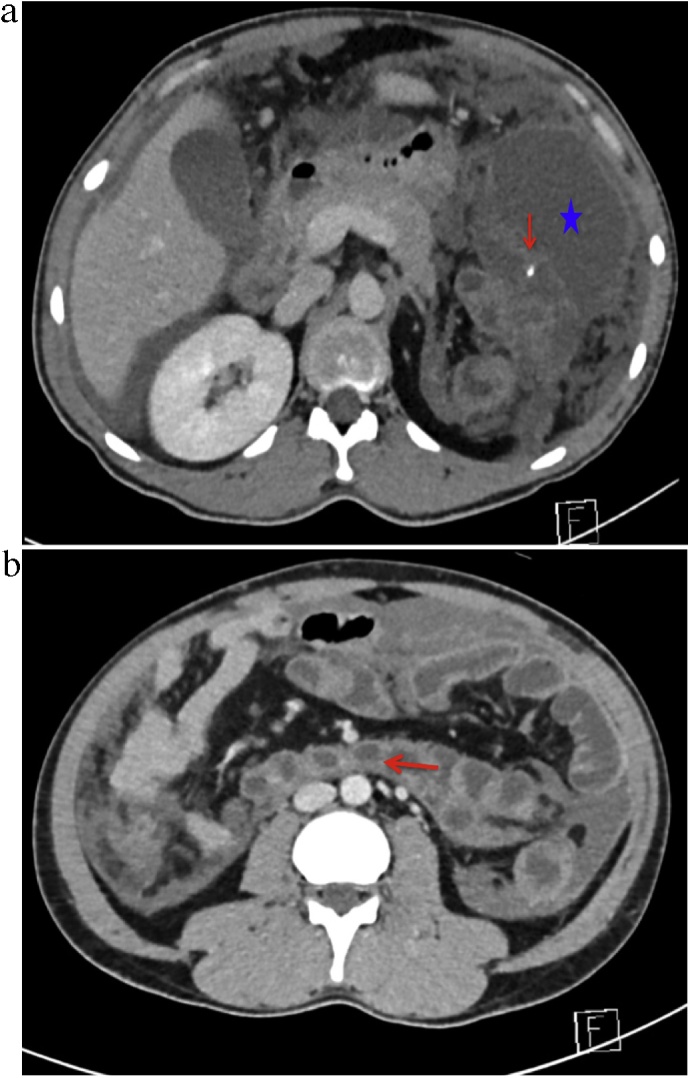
Pre-operative CT images: A) Large phlegmon in LUQ “star” with intra-luminal calcification “arrow”. A large right kidney is also noted. B) Note part of the ascending colon “arrow” is running behind the root of mesentery.
